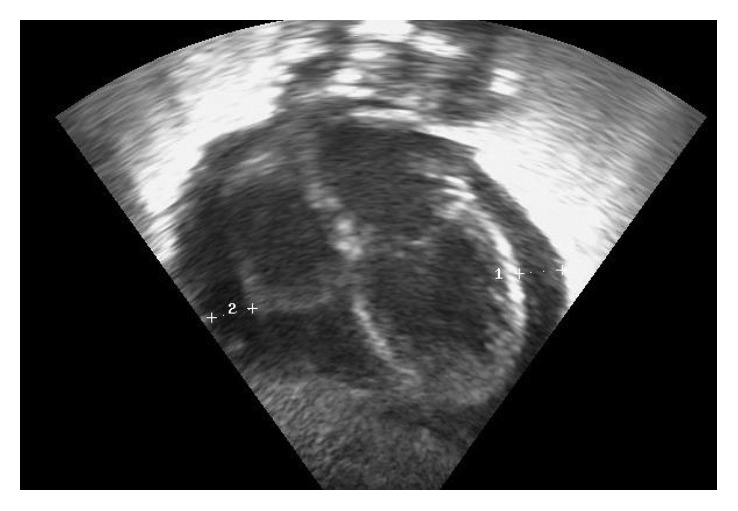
Echocardiography showing pericardial effusion. Dimensions: 1 = 0.43 cm; 2 = 0.41 cm.
